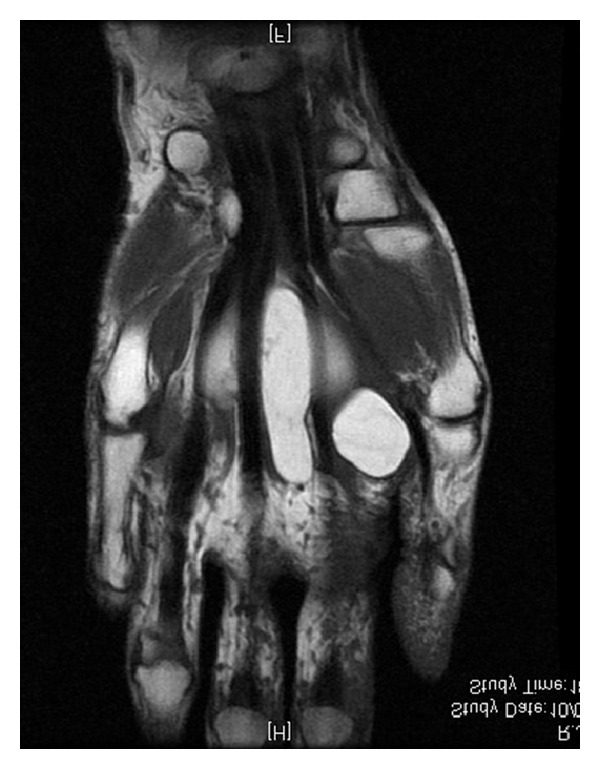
MRI frontal view showing lipoma among flexors tendons and extending to the carpal tunnel.
